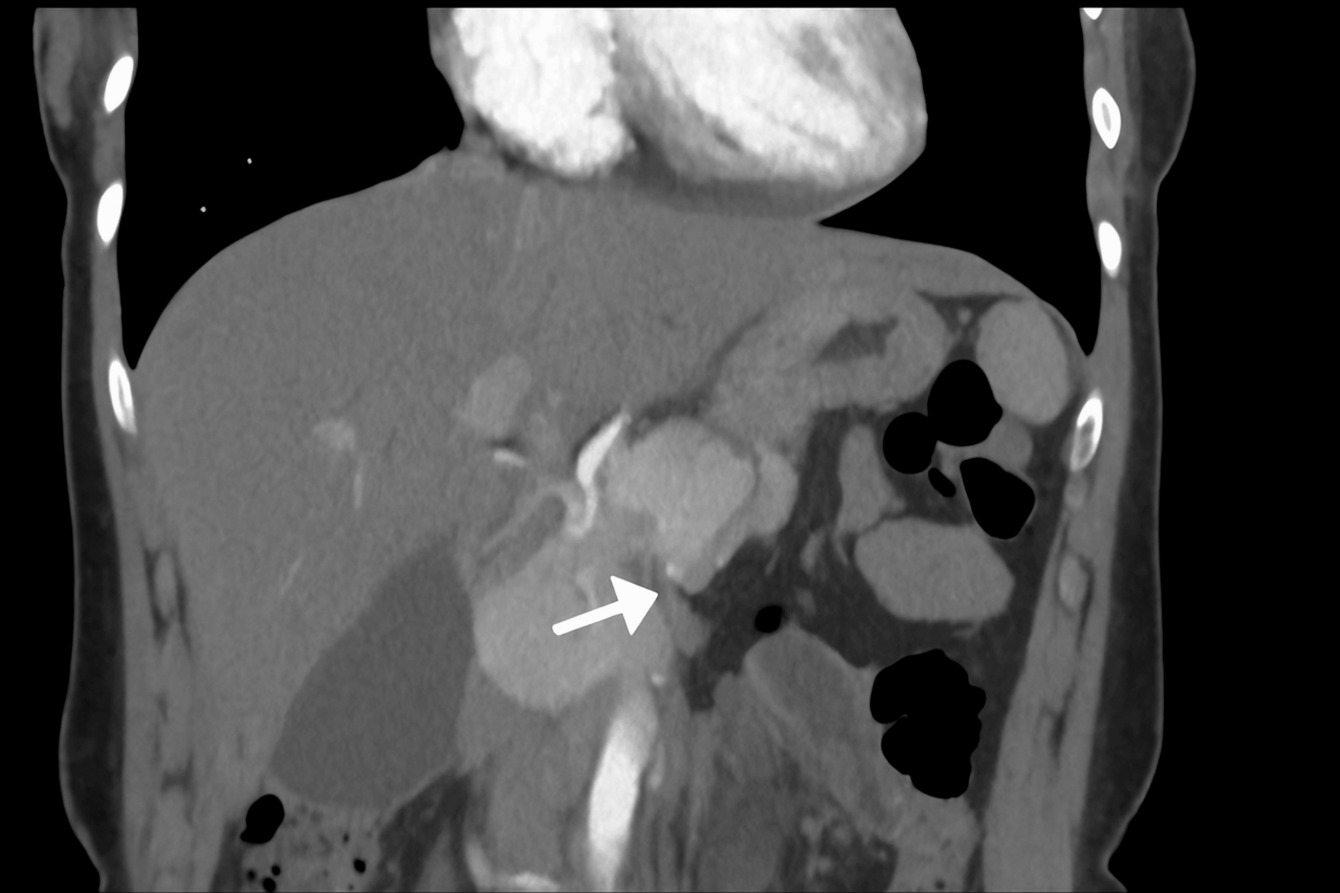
Coronal CT scan demonstrating fluid around the pancreas Another coronal slice reveals mild peripancreatic fluid (white arrow). The surrounding structures appear unremarkable.
